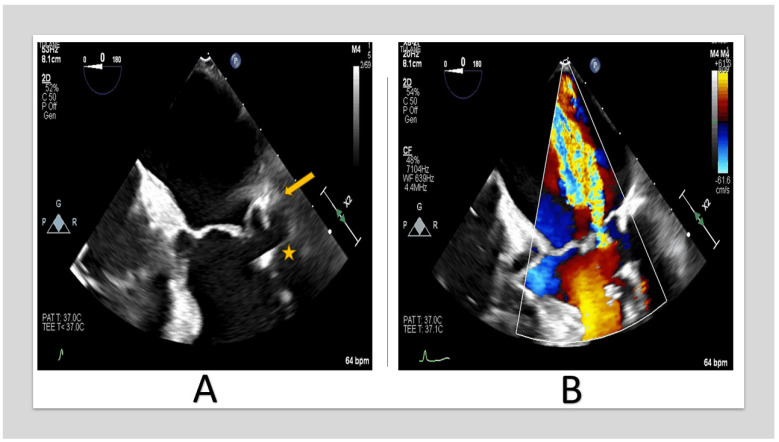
TEE images of 67-year-old woman with severe MR. (A) shows posterior annulus calcification (arrow) with sub-valvular apparatus extension (star. (B) shows posteriorly directed MR jet due to posterior leaflet restriction. (TEE: transesophageal echocardiography, MR: mitral regurgitation).
